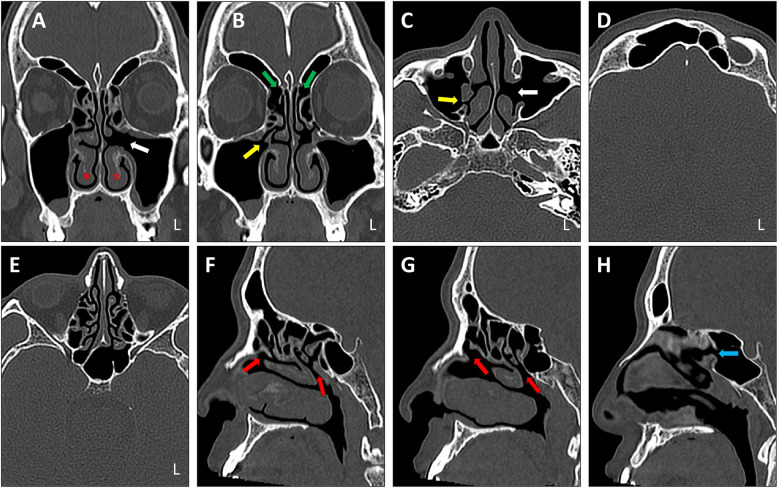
Sinus CT findings of the patient. The key link between headache and sinusitis lies in whether the drainage pathway is obstructed. In this patient, the sinus CT showed no definitive evidence of impaired drainage; therefore, the imaging findings do not support a confirmed diagnosis of sinusitis-related headache. (A–D) Postoperative changes are observed in the left maxillary sinus, with a widened ostium (white arrows in A, C); marked thickening of the sinus mucosa was observed on the medial, superior, and inferior walls of the left maxillary sinus, with partial nodular appearance; a small amount of fluid level was noted in the right maxillary sinus, with unobstructed ostium (yellow arrows in B, C); bilateral inferior turbinate hypertrophy was present (red asterisk in A), and the nasal cavity was basically unobstructed; bilateral frontal sinuses developed well (D), without fluid level in the sinuses; no mucosal hypertrophy, mucus retention, cysts, polyps were found in the frontal sinus ostium/frontal recess area, and the ostium was unobstructed (green arrow in B). (E–G) Bilateral ethmoid air cells developed well with intact bony septa, and mild thickening of the bilateral ethmoid sinus mucosa was seen (E); the openings of the anterior and posterior ethmoid air cells were unobstructed (red arrows: F for left ethmoid sinus, G for right). (H) The sphenoid sinus was well pneumatized without mucosal thickening; the sphenoid ostium was unobstructed, and no soft tissue obstruction was observed (blue arrow).
